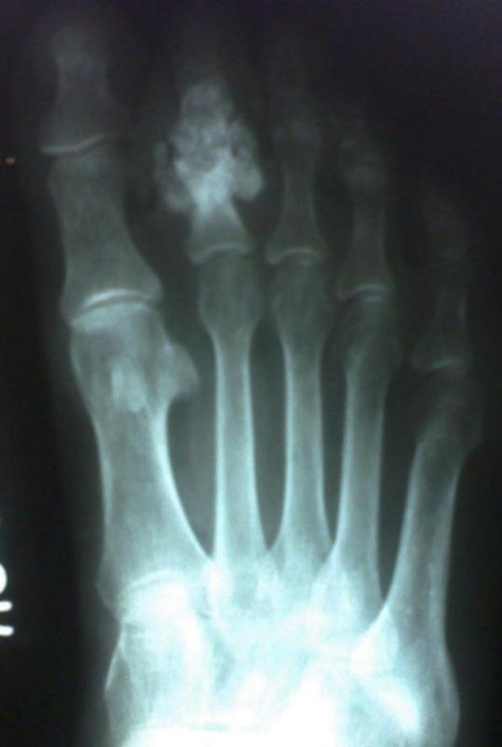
Preoperative radiograph of the foot.
